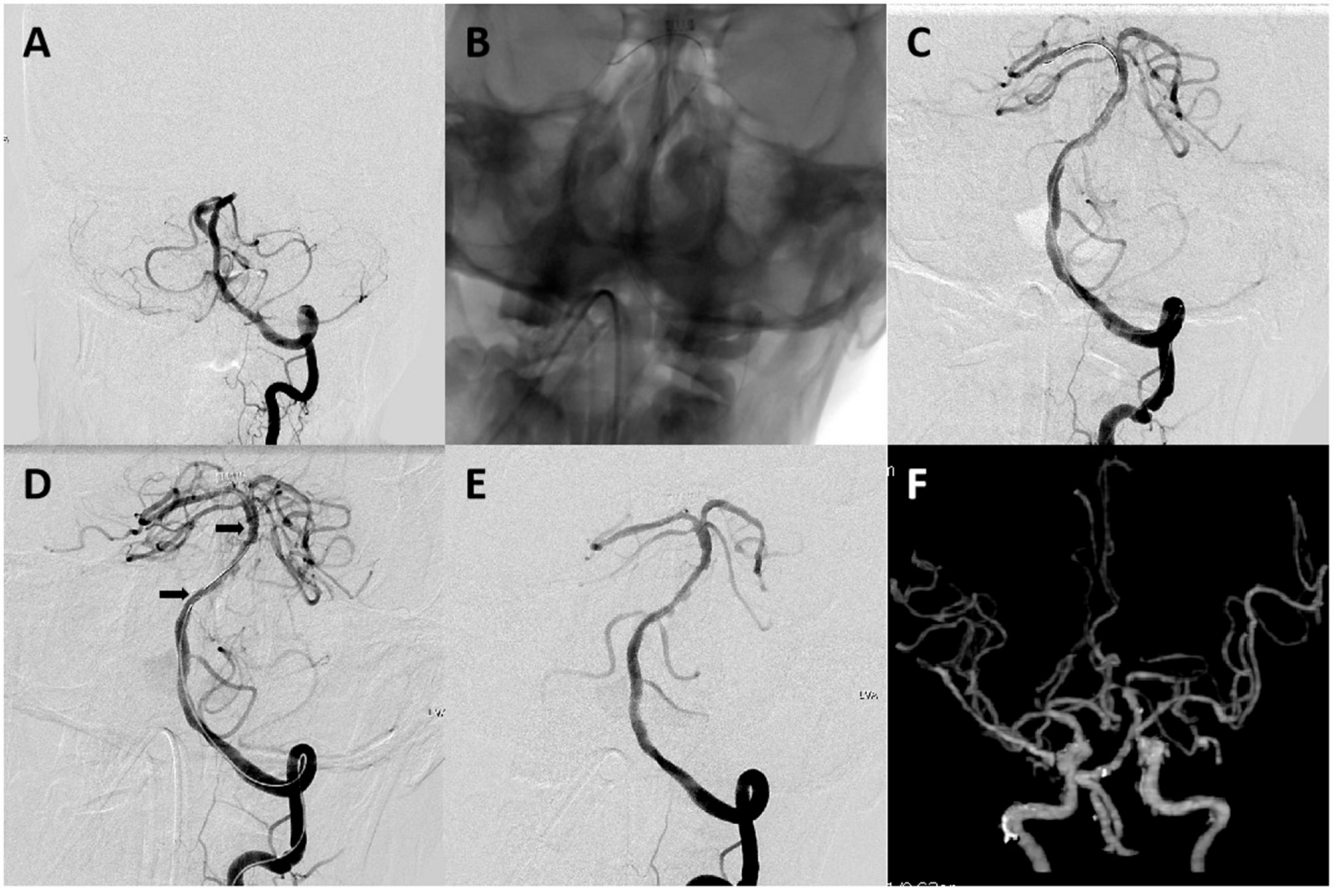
A 64-year-old male patient with acute stroke due to acute basilar artery occlusion. (A) Left vertebral artery angiogram revealed occlusion at the proximal basilar artery. (B) A 2 mm × 15 mm balloon (gateway) was deployed and inflated in the occluded segment. (C–E) Left vertebral artery angiogram obtained after intracranial angioplasty and stent placement revealed complete basilar artery recanalization and good distal perfusion. Arrows indicate proximal and distal ends of the Enterprise stent (4 mm × 22 mm). (F) CT angiography on 24-h follow-up revealed a patent basilar artery. The patient had a mRS score of 1 on a 3-month follow-up.
